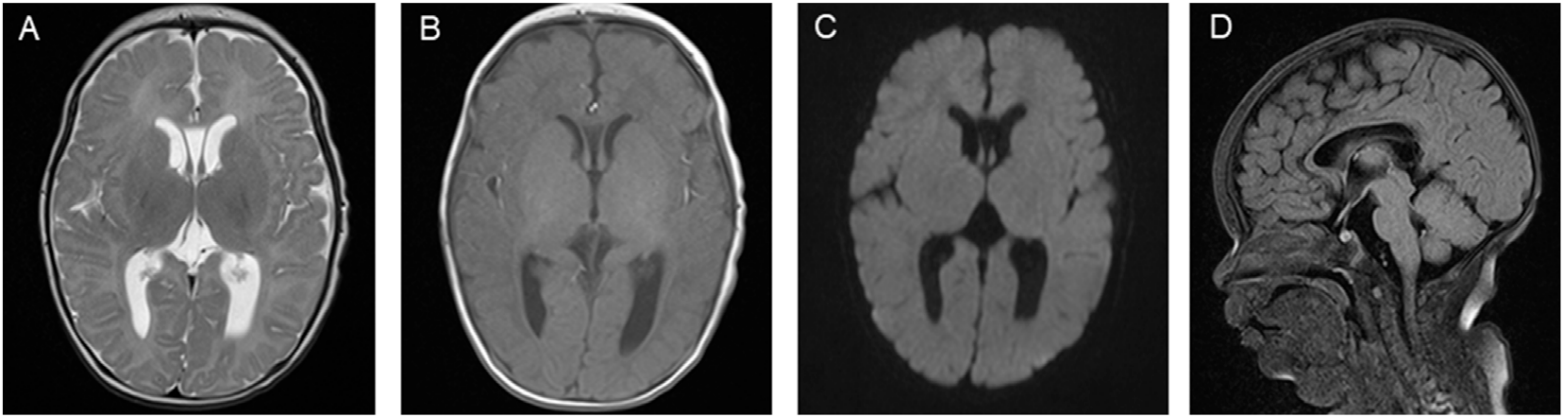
Brain magnetic resonance imaging (MRI) images on the patient’s 51st day of life. (A) T2-weighted imaging (T2WI) showing symmetrical bilateral cerebral hemispheres with normal gray and white matter signal intensities. (B) T1-weighted imaging (T1WI) showing high signal intensity in the posterior limb of the internal capsule and the cavum septum pellucidum. (C) Diffusion-weighted imaging (DWI) showing no obvious abnormal signal. (D) Fluid-attenuated inversion recovery (FLAIR) sequence showing the corpus callosum genu and body with normal morphology.
